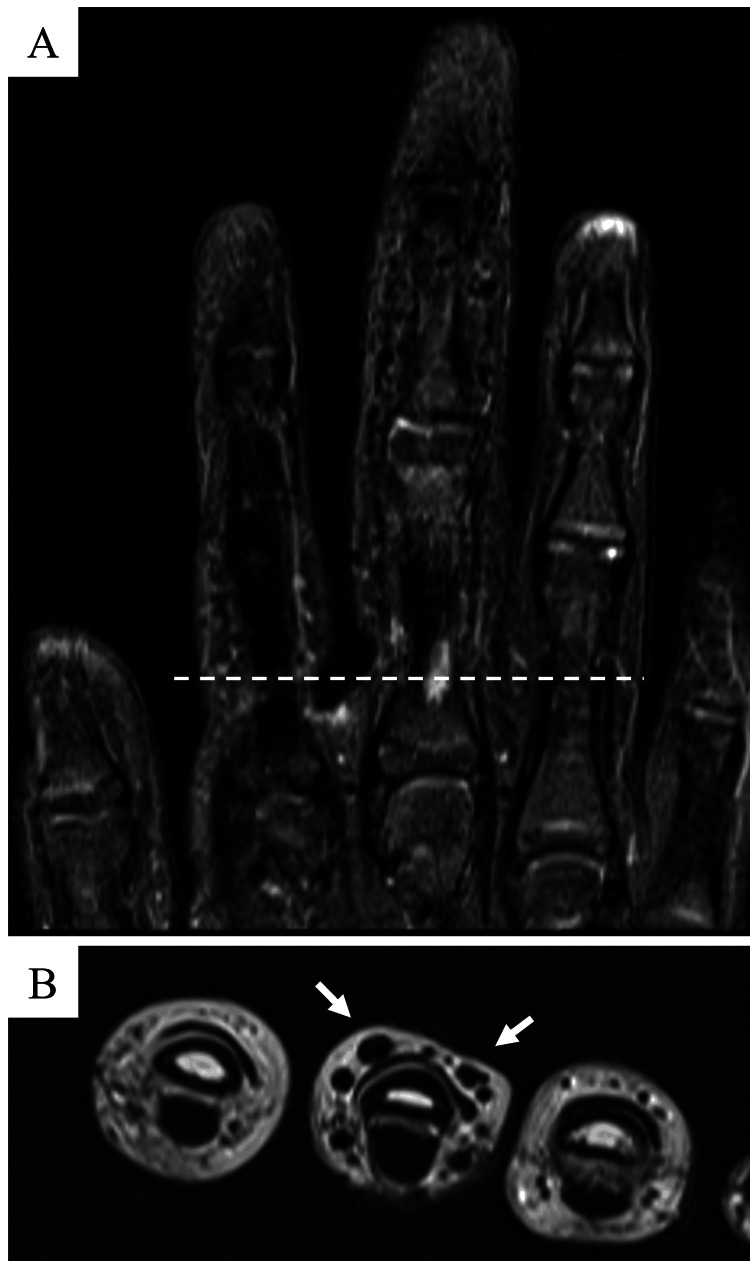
Magnetic resonance imaging of the patient’s finger. (A) Coronal image (fat-suppressed T2-weighted) of right fingers. (B) Axial image (T2-weighted) of right fingers. Magnetic resonance imaging revealed dilation of the arteries and veins, but no tumor was detected.
